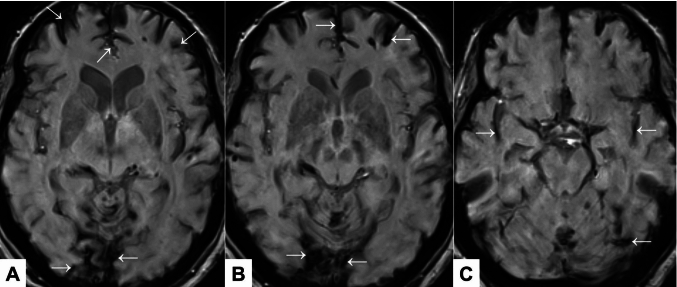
Axial susceptibility-weighted magnetic resonance images of supratentorial involvement (Case 4). Appearances of hypointense regions (cephalad to caudal, A–C) suggestive of hemosiderin deposits are marked with arrows. Source: UK Biobank ©. Reproduced by kind permission of UK Biobank ©
